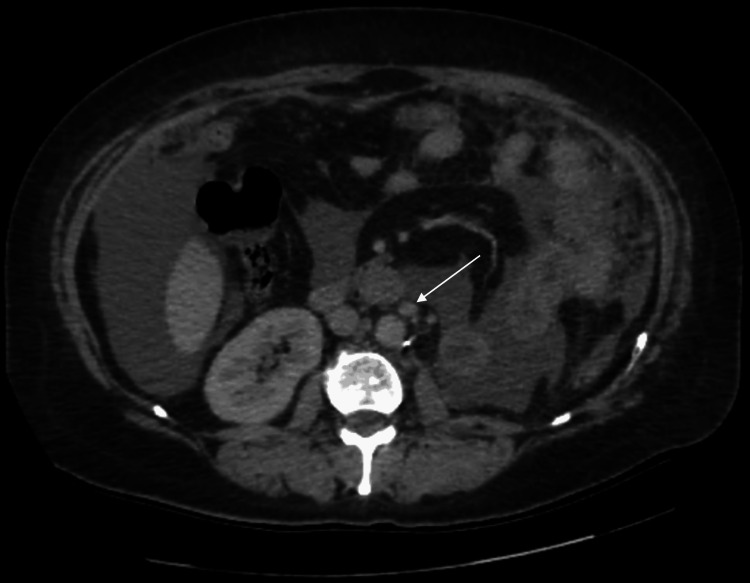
Axial CT scan showing preaortic lymph nodes
